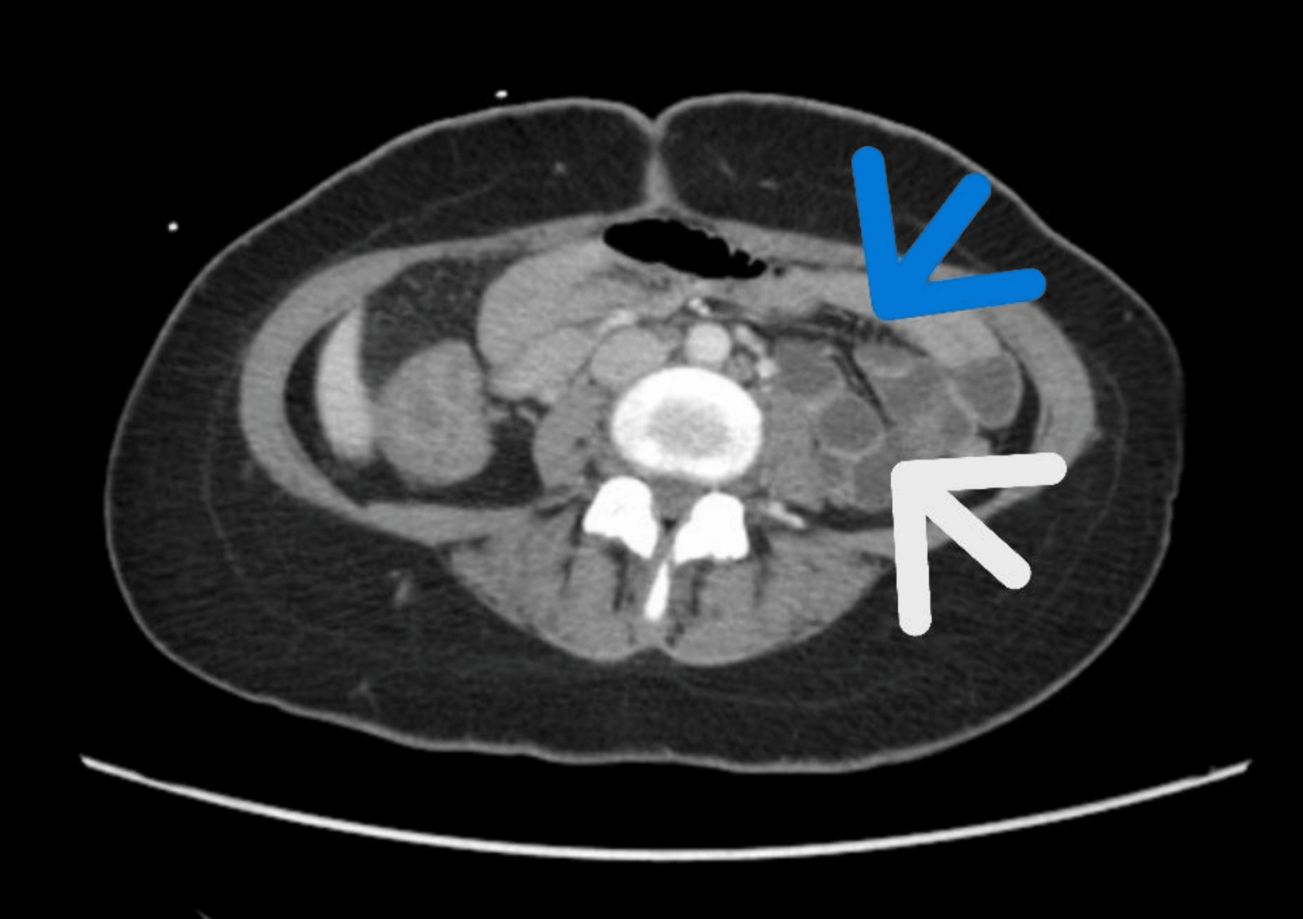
CT Abdomen/Pelvis: Diffuse inflammatory changes of the colon Blue arrow points to fat stranding. White arrow points to wall thickening.
